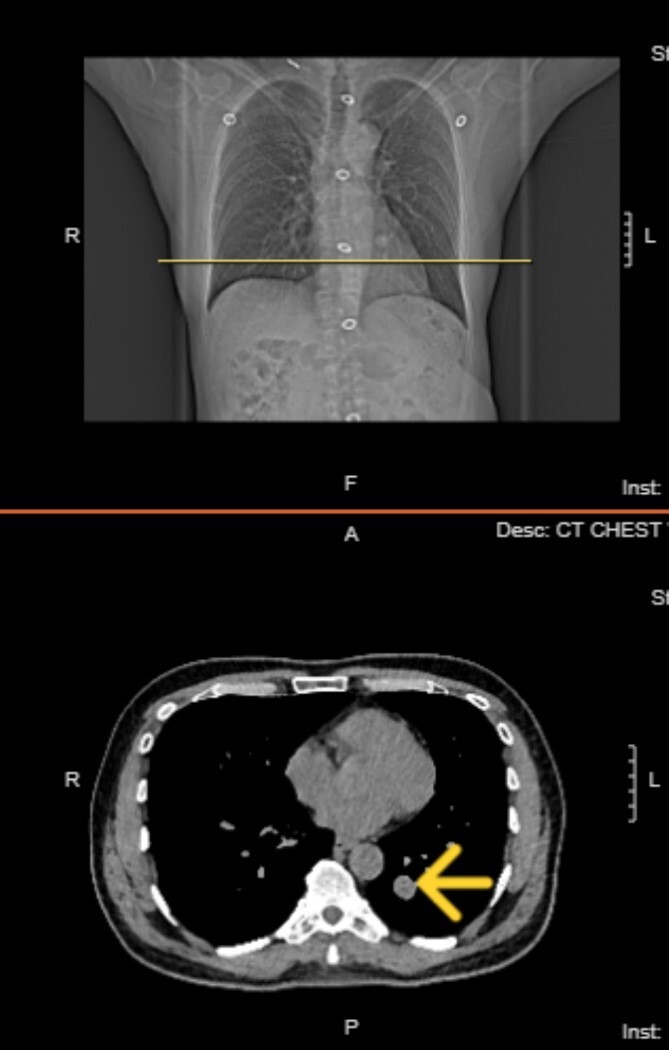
Top image: Coronal view indicating the level of the solitary fibrous tumor (SFT). Bottom image: Axial CT scan at the indicated level, showing a well-circumscribed, homogeneous mass.
